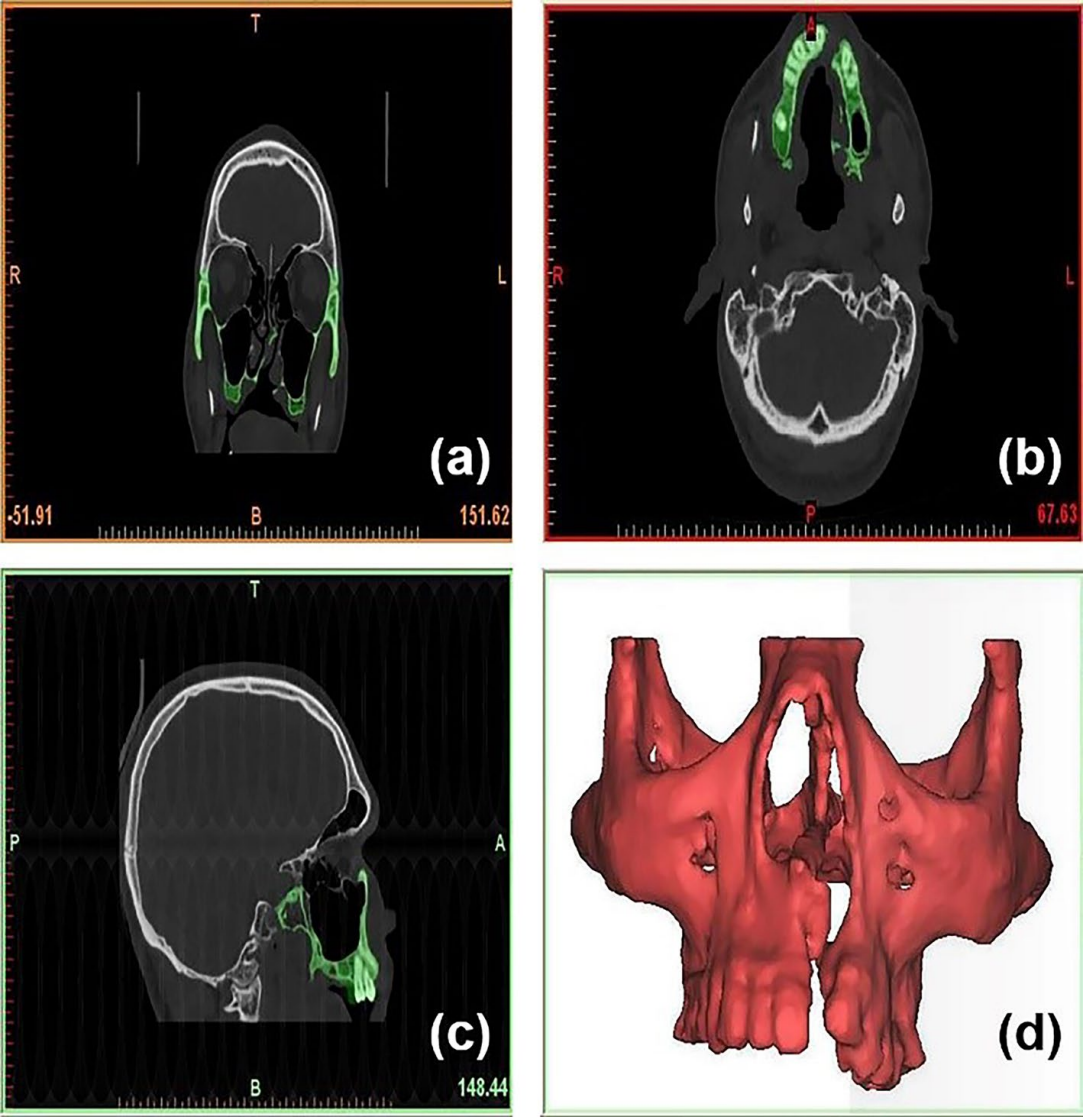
CT scan images from a axial, b coronal, c sagittal plane and d 3-D model of maxillary complex of a unilateral cleft lip and palate
